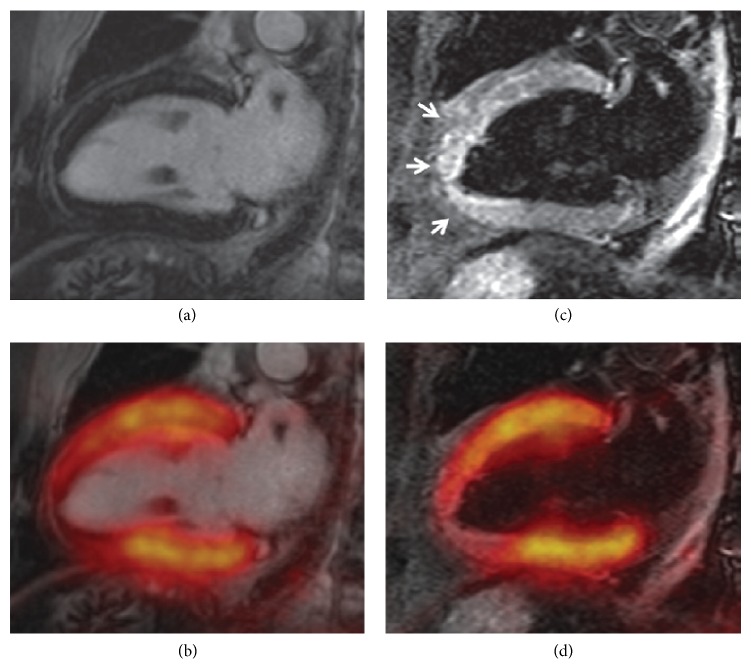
Cardiac PET/MR imaging of a 66-year-old male patient with ST-elevation myocardial infarction and acute occlusion of the left anterior descending artery. (a) Late gadolinium-enhanced (LGE) image shows no infarction zone. (b) Fused LGE and PET images show that tracer uptake was reduced. (c) MR image shows myocardial edema (arrows) that corresponded well with the area of reduced tracer uptake on (d) (from [118] with permission).
